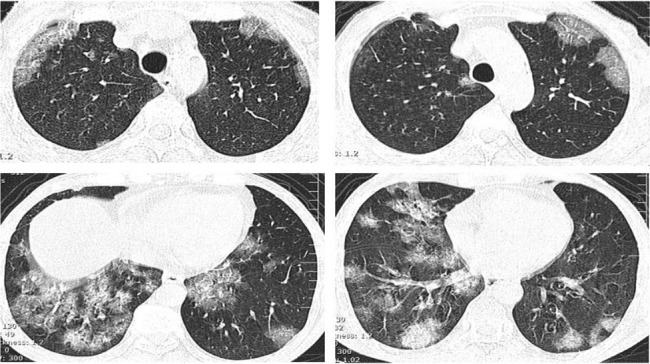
Chest CT shows Crazy-paving pattern and ground glass opacity with lower lobes and peripheral distribution.
